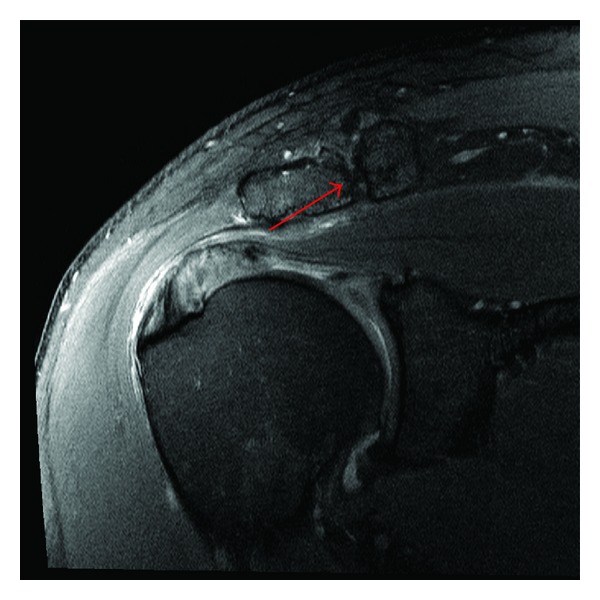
Coronal PD SPAIR magnetic resonance image of right shoulder showing moderate-to-severe osteoarthritis of the acromioclavicular joint (arrow), without any conflicting osteophytes in regard to the underlying rotator cuff.
